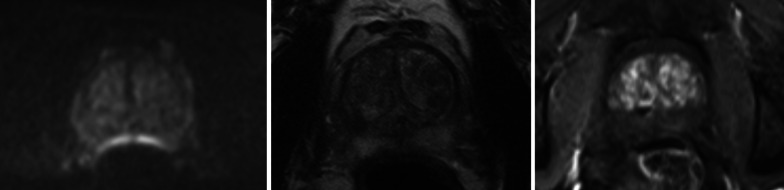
66 year-old patient undergoing prostate MRI for suspicion for prostate cancer. The presence of rectal gas results in susceptibility artifacts, distorting the diffusion-weighted image (left: DWI, *b* = 1000 s/mm^2^; middle: *T*2—weighted). Both the experienced radiologists and the AI opted for contrast, while reader 3 (technician) did not. Dynamic contrast-enhanced MRI (right) was helpful in ruling out any index lesions at this level of the prostate
